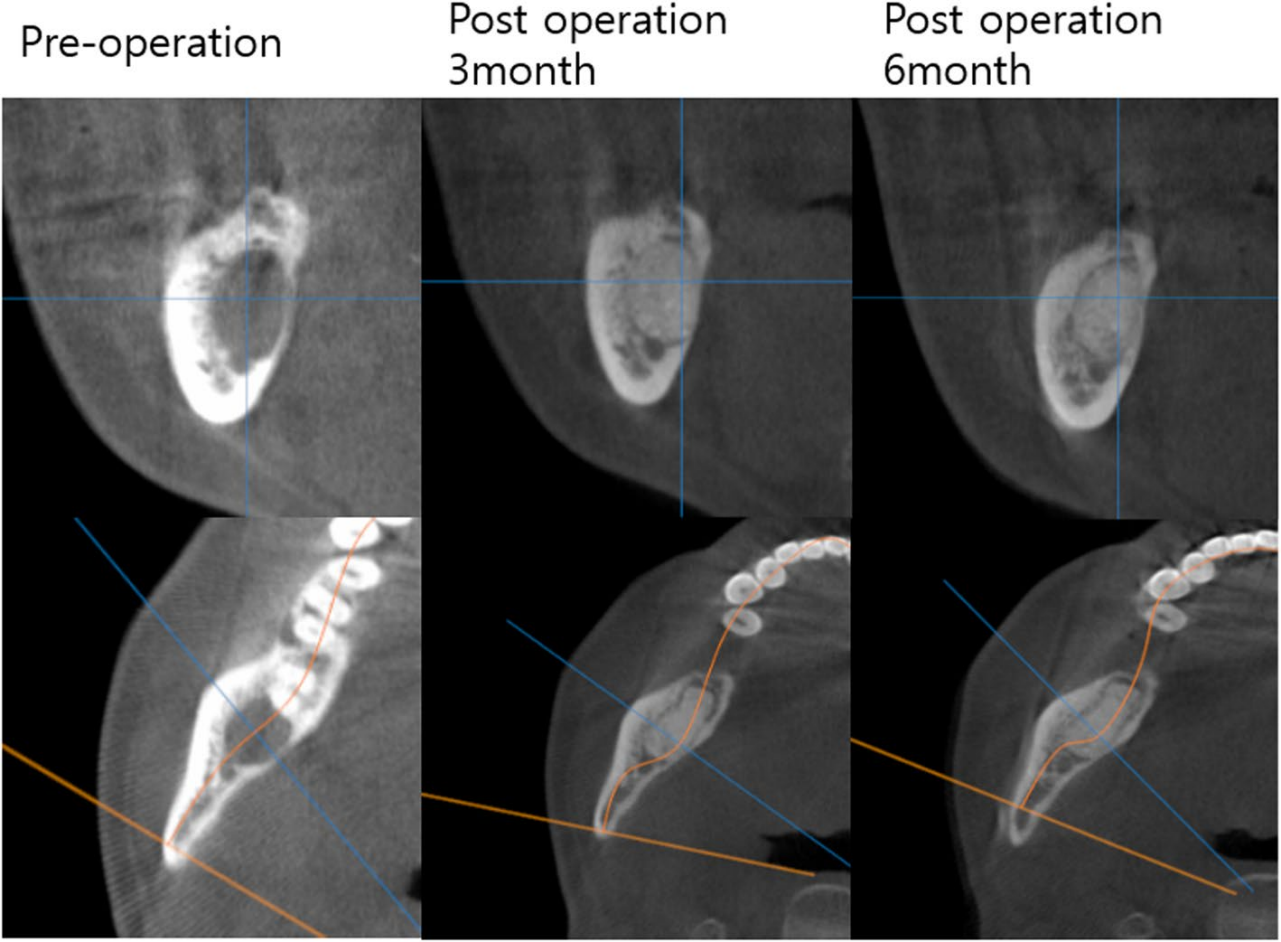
Preoperative and postoperative cone beam CT
